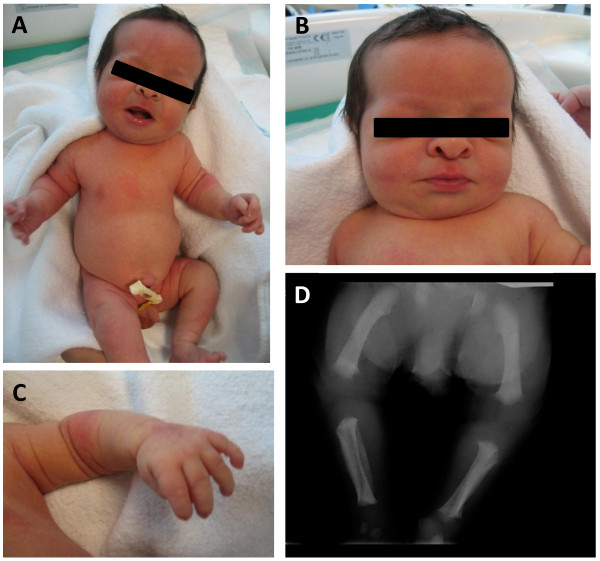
Clinical and radiological findings. A. Patients general appearance with rhizomelic limb shortening. B. A large head with protruding forehead, mid facial hypoplasia, depressed nasal bridge, hypoplasic chin, short neck. C. Short trident hands. D. Radiograph showing bowing and shortness of legs with metaphyseal flaring.
